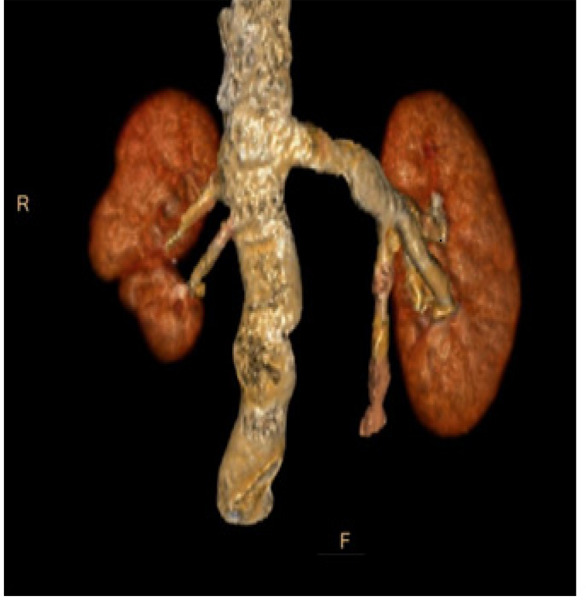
3D reconstruction of CT urography image in venous phase showed a separate vein from right supernumerary kidney draining into the inferior vena cava.
